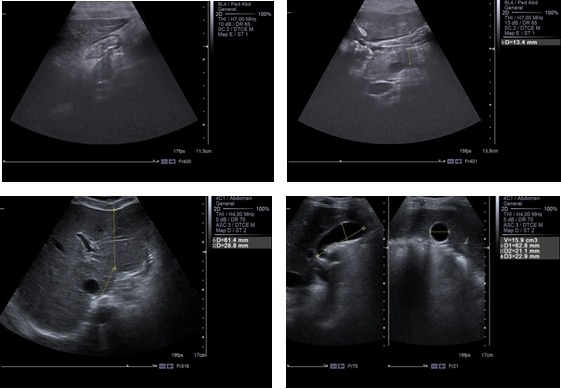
Important aspects of abdominal ultrasonography at admission in our unit
